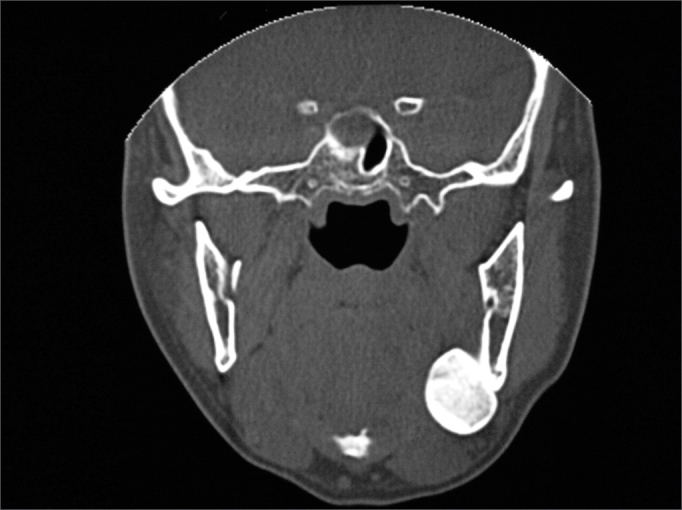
Preoperative aspect: Coronal CT scan showing a peripheral osteoma in the left mandible angle.
